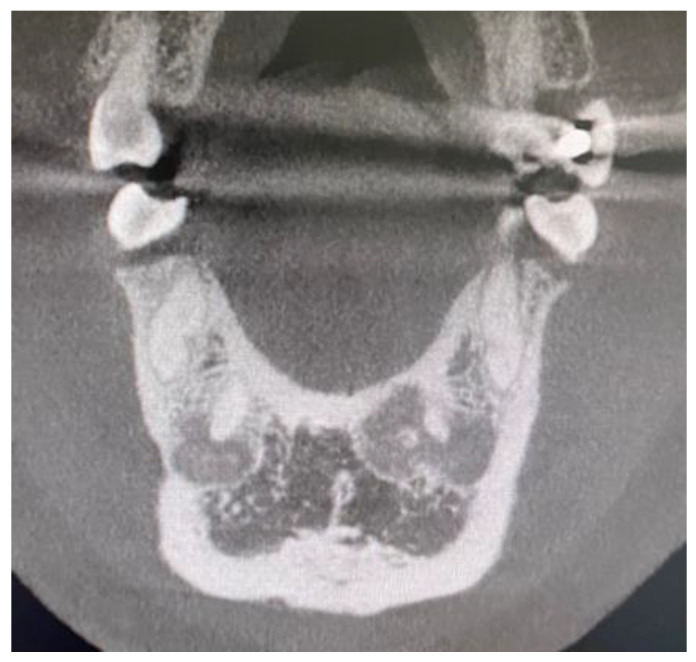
CBCT, coronal view, COD surrounding the periapical area of the mandibular incisors.
